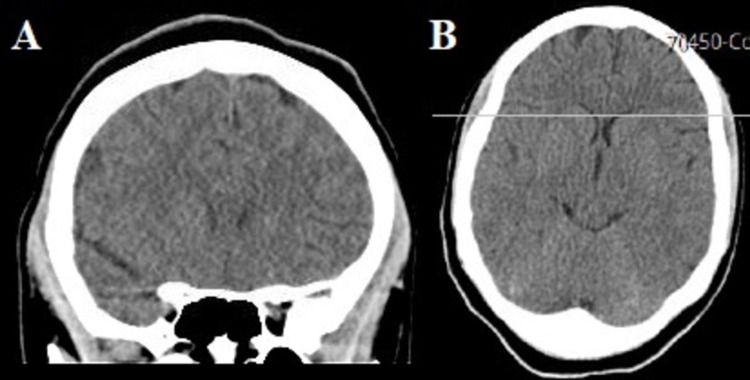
Radiological disappearance of subdural hematoma Noncontrast coronal (A) and axial (B) cranial CT scan showing complete resolution of the small left subdural hematoma.
